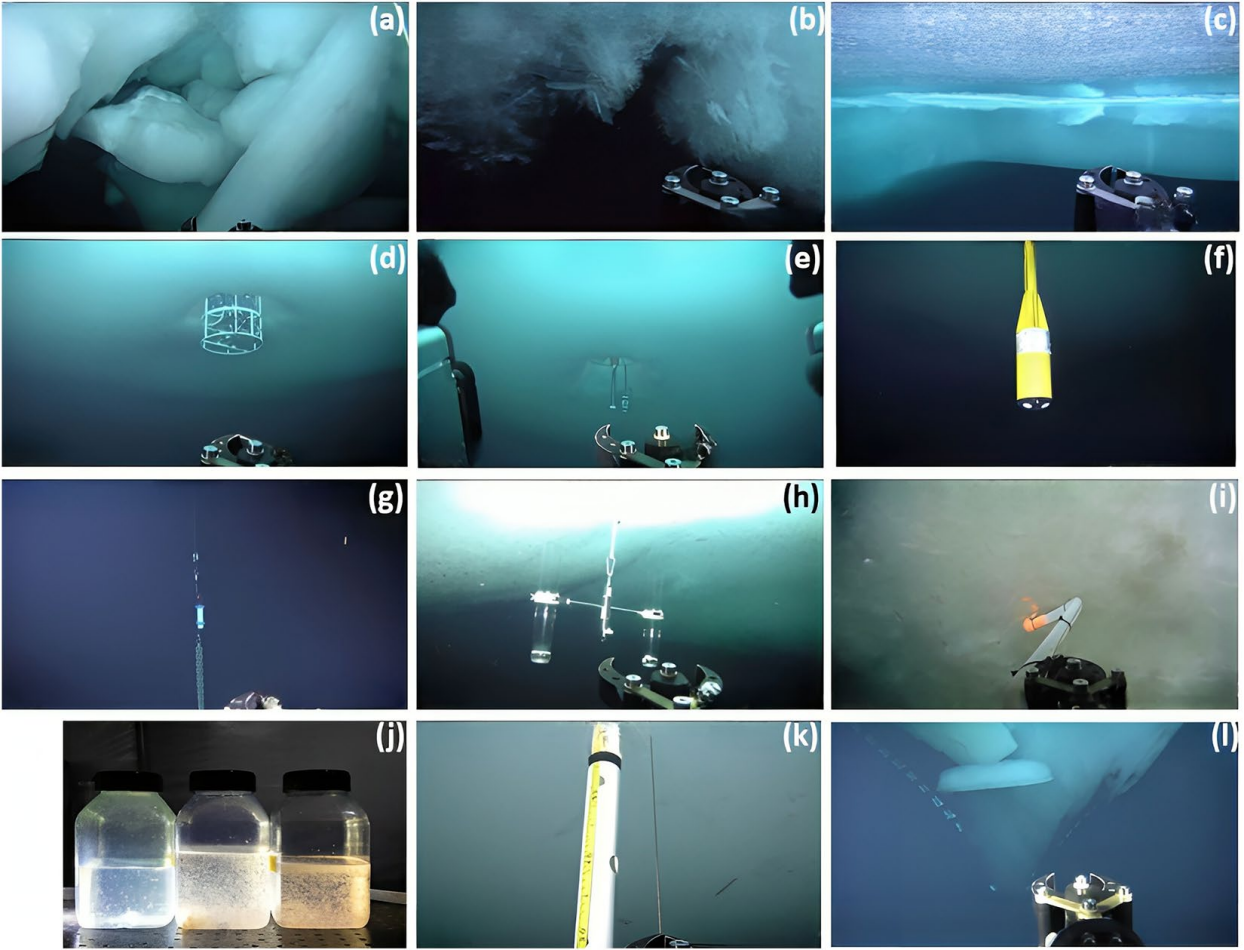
(a) Filming ridged sea ice and observe ice-block sizes, (b) documenting platelet ice and (c) new ice formation, observing (d) a CTD cast and (e) a Micro Structure Sonde cast at Ocean City, documenting a permanent (f) Acoustic Doppler Current Profiler and (g) CTD installation, (h) inspecting a sediment trap, (i) sampling of algae by a suction pump, (j) photographs of zooplankton catches from three different depths on 08/02/2020, (k) video screenshot of reading sea-ice thickness from stakes, and (l) inspecting the hull of *Polarstern*.
